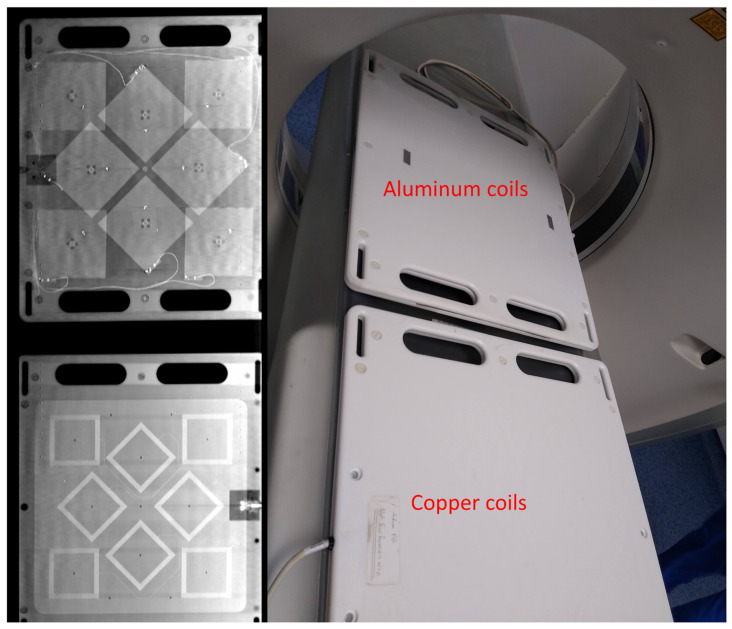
A direct comparison of the two different field generator designs. Both devices were put through the CT scanner together to allow for easier comparisons. From the aluminum coils, the FR4 substrate is visible but the actual coils cannot be discerned. This is in stark contrast to the copper coils which can be clearly identified.
